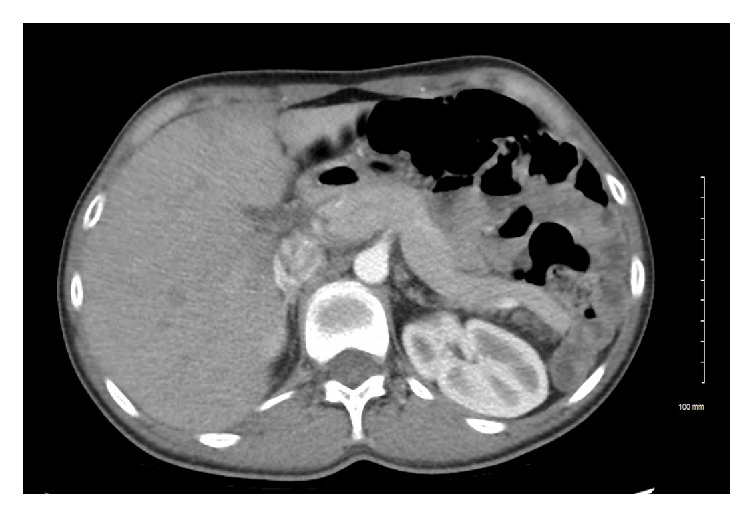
Axial view CT of the abdomen showing elongated pancreatic tail.
